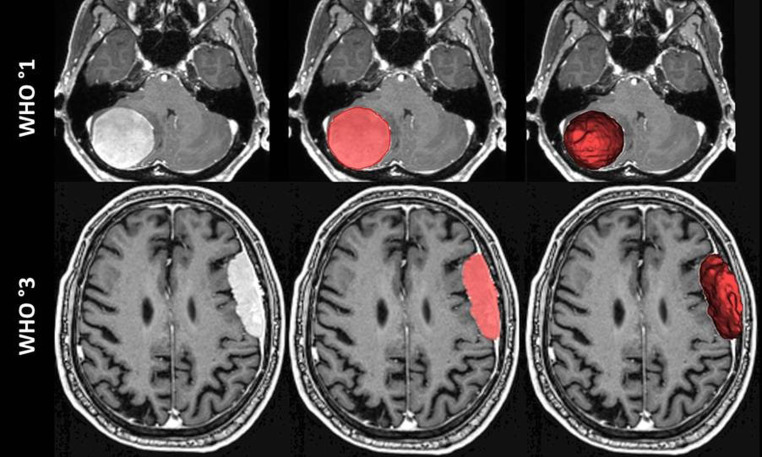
Non-invasive analysis of brain tumor to aid in accurate tumor grading. Axial reformat of a T1w post-Gd MRI of a right cerebellar benign meningioma (top row) and a left frontal anaplastic meningioma (bottom row) with unprocessed data on the left, tumor segmentation of the respective slice in the middle and superimposed 3D tumor segmentation on the right. The grade 1 meningioma demonstrates a regular, rounded shape with higher SR and sphericity. In contrast, the grade 3 meningioma exhibits an irregular, elongated and less round shape, accompanied by lower SR and sphericity
